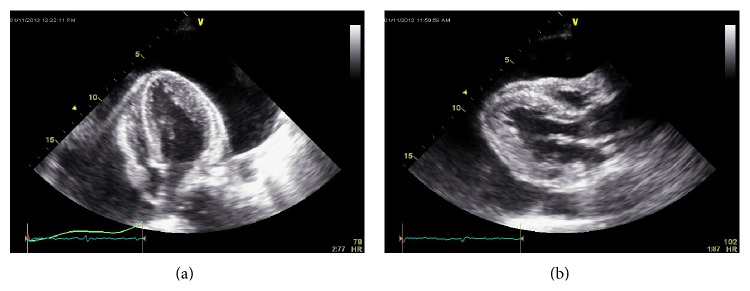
Echocardiogram. (a) Apical four-chamber view showing “swinging heart” and large pericardial effusion, (b) parasternal long axis view showing right ventricular diastolic collapse.
